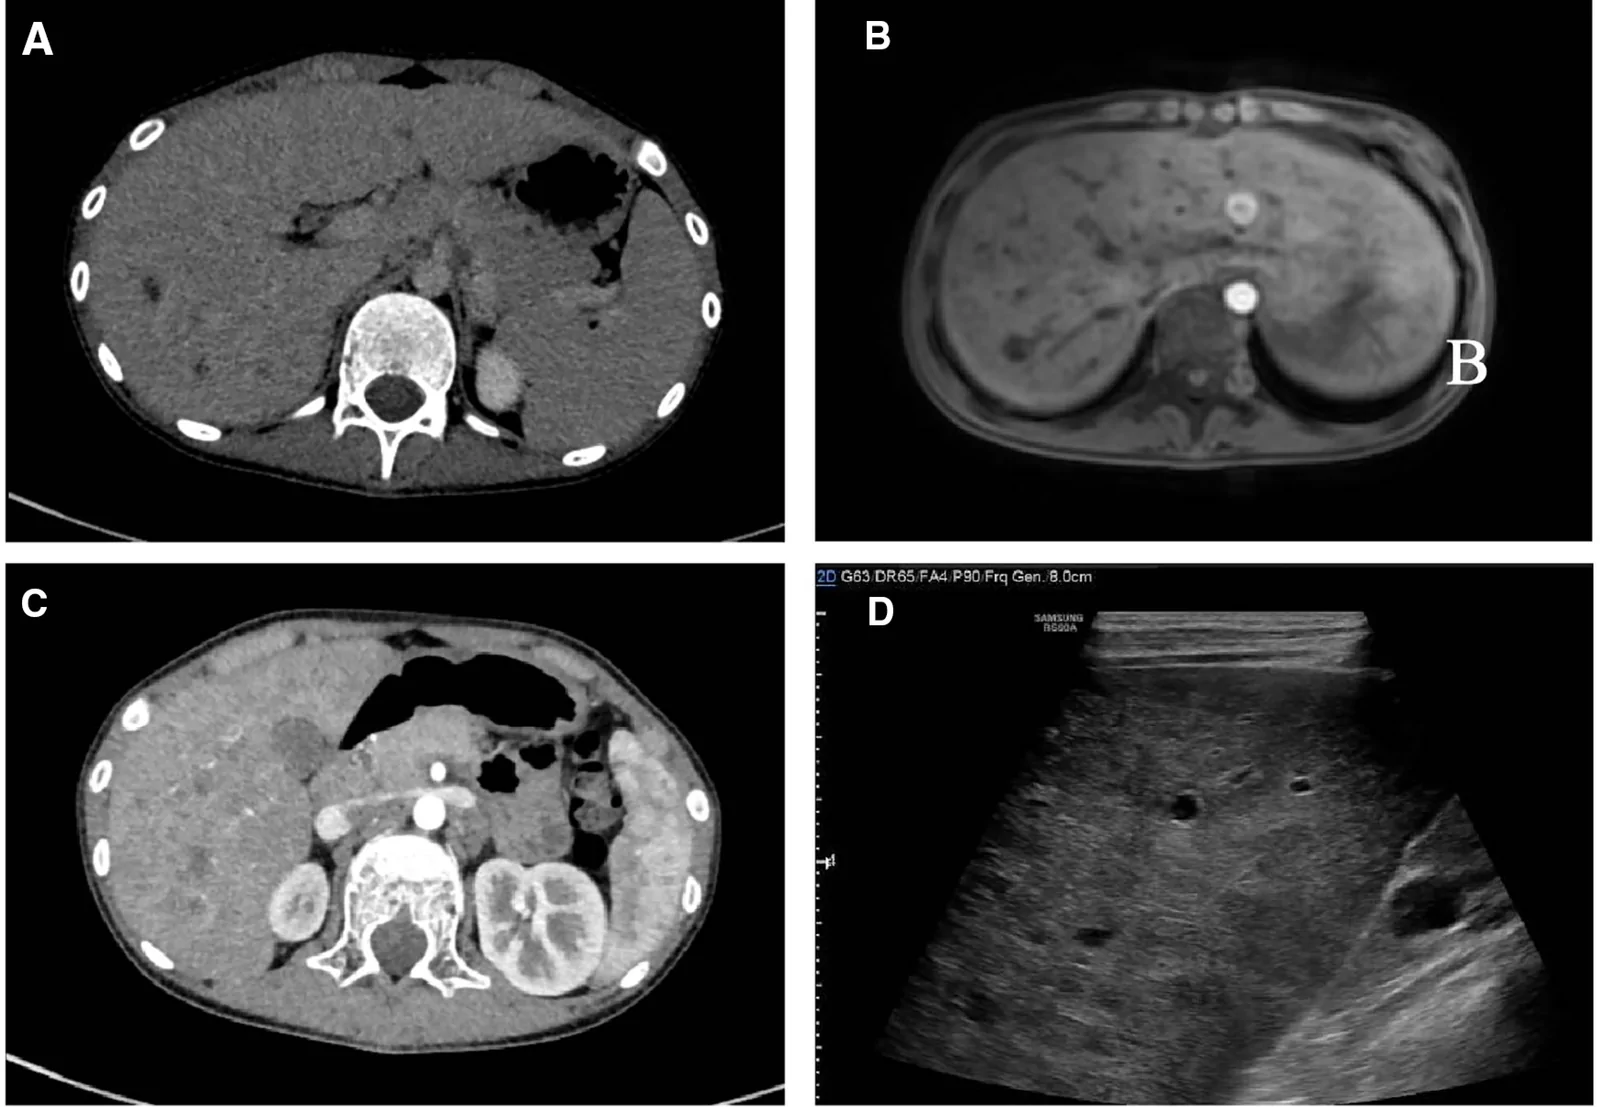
Imaging findings of liver lesions. (A) CT showed multiple circular hypodense shadows in the liver parenchyma with unclear boundaries. (B) Enhanced CT in the arterial phase showed mild enhancement. (C) Enhanced MRI showed multiple enhanced lesions in the liver parenchyma. (D) Two-dimensional ultrasound image of the liver showed multiple hypoechoic nodules with clear boundaries. CT = computed tomography, MRI = magnetic resonance imaging.
